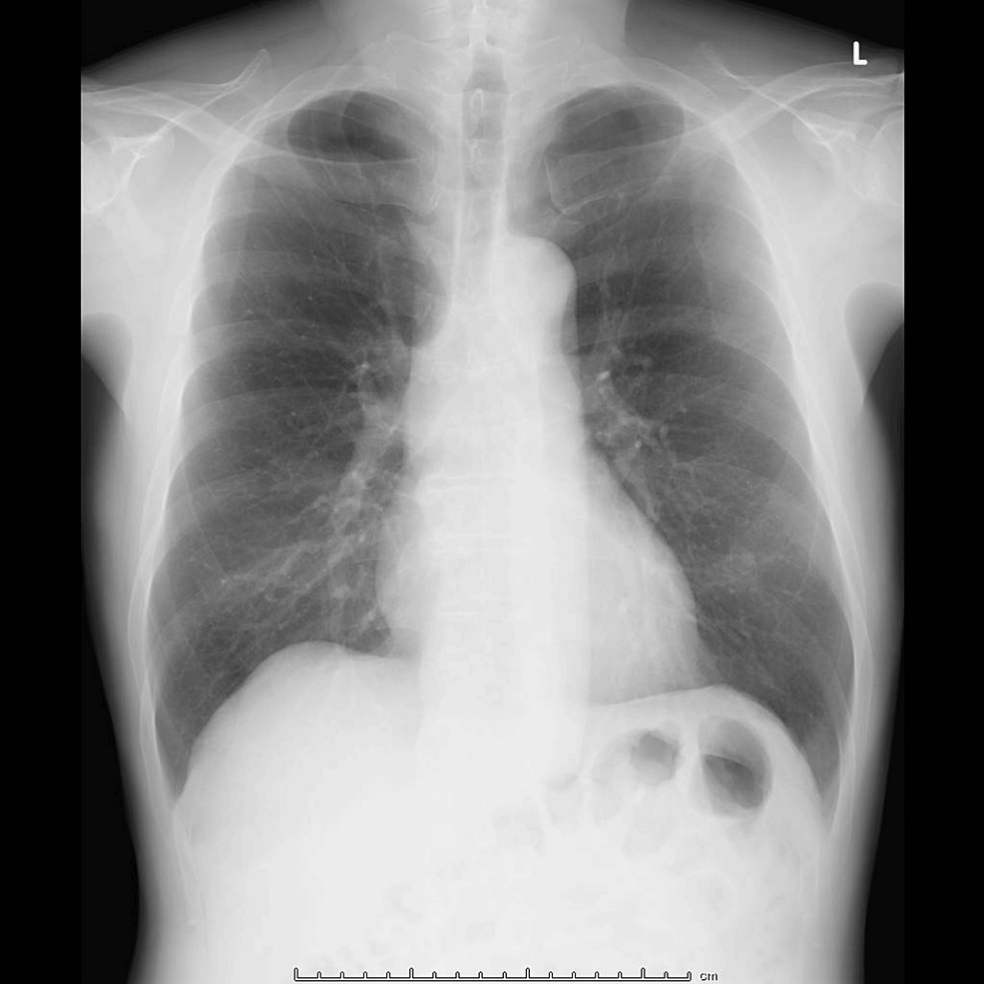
Preoperative chest X-ray. Intestinal gas was observed under the left diaphragm.
